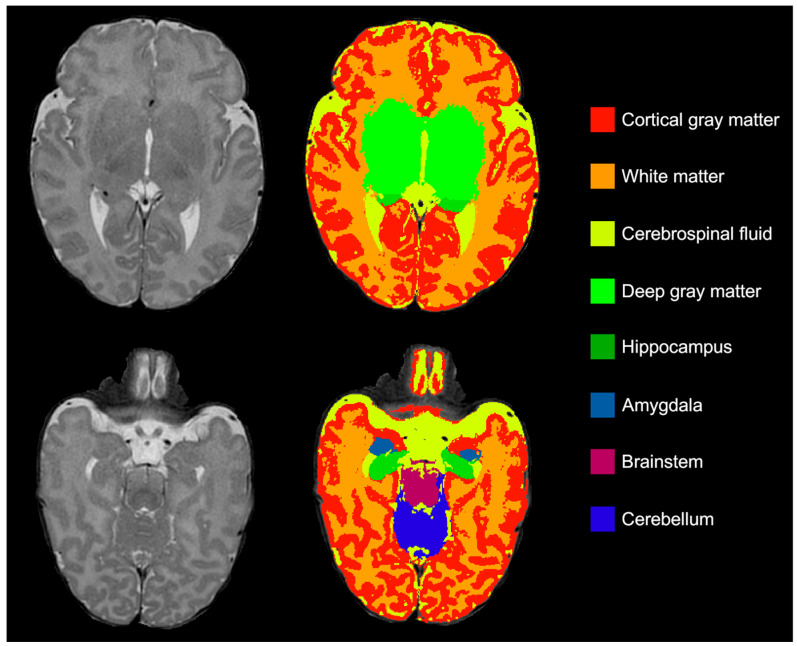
T2-weighed MRI (left) alongside color-coded segmented brain volumes (right).
